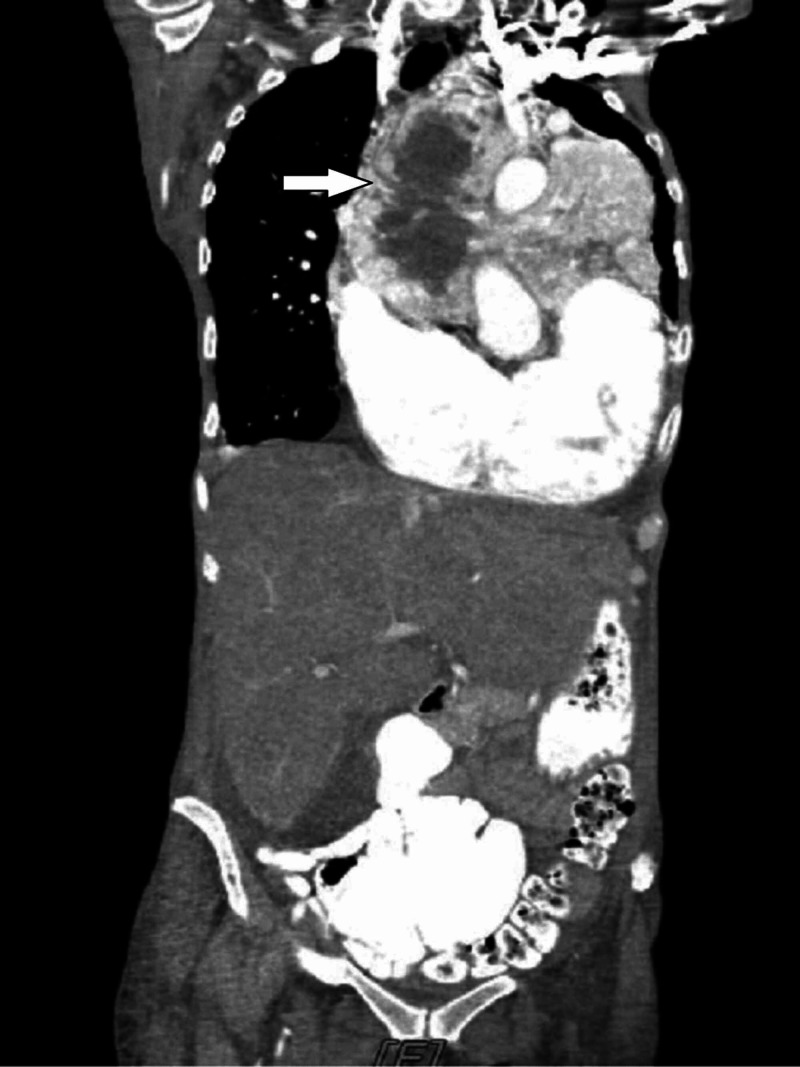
CT scan: coronal section of the chest and abdomen shows a large upper anterior mediastinal mass, displacing most of the left lung parenchyma, with mass effect on the heart
